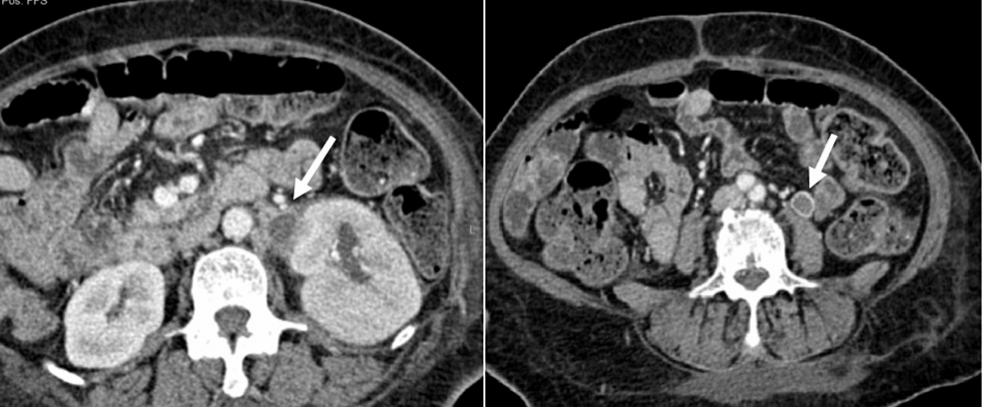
Post-contrast CT images (axial) Two axial post-contrast CT images demonstrate a thrombosed left ovarian vein (white arrows) due to left pyelonephritis in a 41-year-old diabetic female patient treated by the authors at King Abdulaziz University Hospital.
